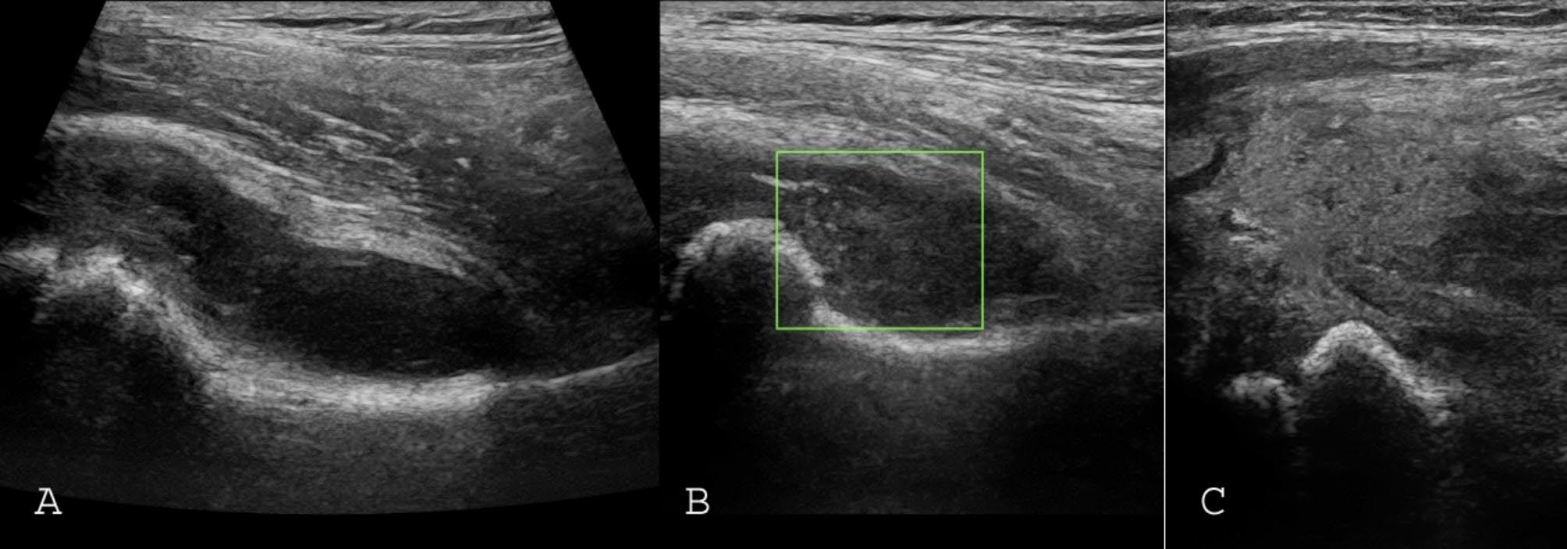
The sonographic sign in three patients with late diagnosed septic arthritis: (A) Septated effusion. (B) Hip effusion with massive debris (no-vessels on Doppler color box differentiates it from synovial hypertrophy) (C) Joint capsule perforation and pus extension to extra- arthicular space
